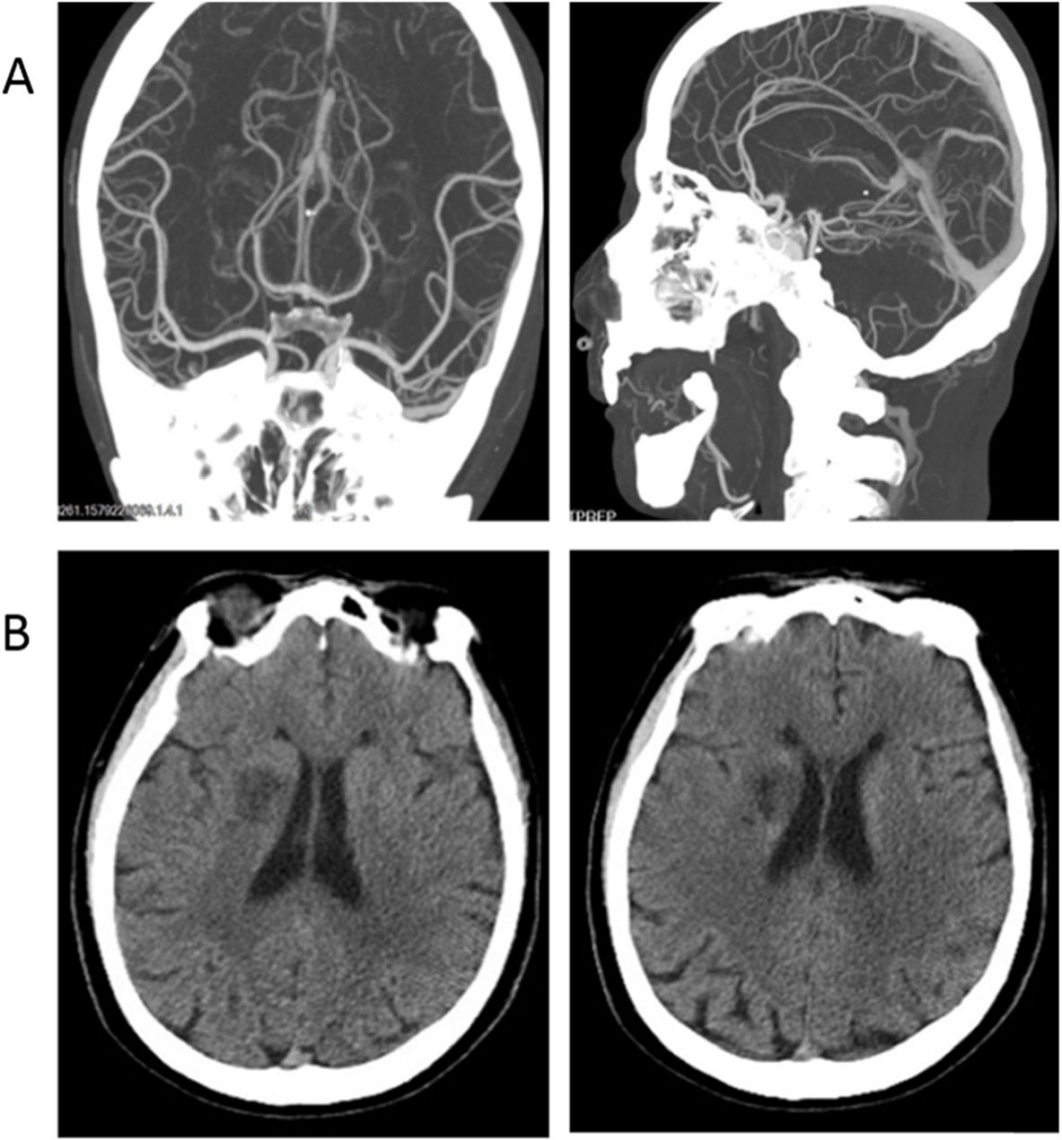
The head/neck atrial CTA and head CT of case 2 infected with SARS-CoV-2. a: Head CTA on January 17, 2020, routine surgical evaluation before thyroid cancer removal surgery, b: Head CT on February 10, 2020, Illness day 9
